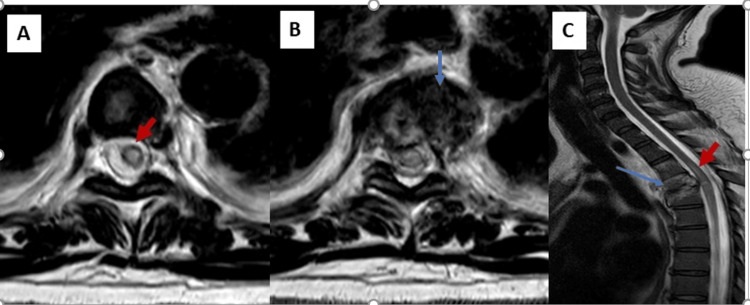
MRI T2 sequence in axial (A, B) and sagittal (C) views Displaced compression fracture of the fourth dorsal vertebrae on the left paravertebral side (blue arrow), causing posterior wall retraction, reducing the subarachnoid spaces, and compressing the adjacent spinal cord, with T2 hyperintensity indicating a spinal cord injury (red arrow)
